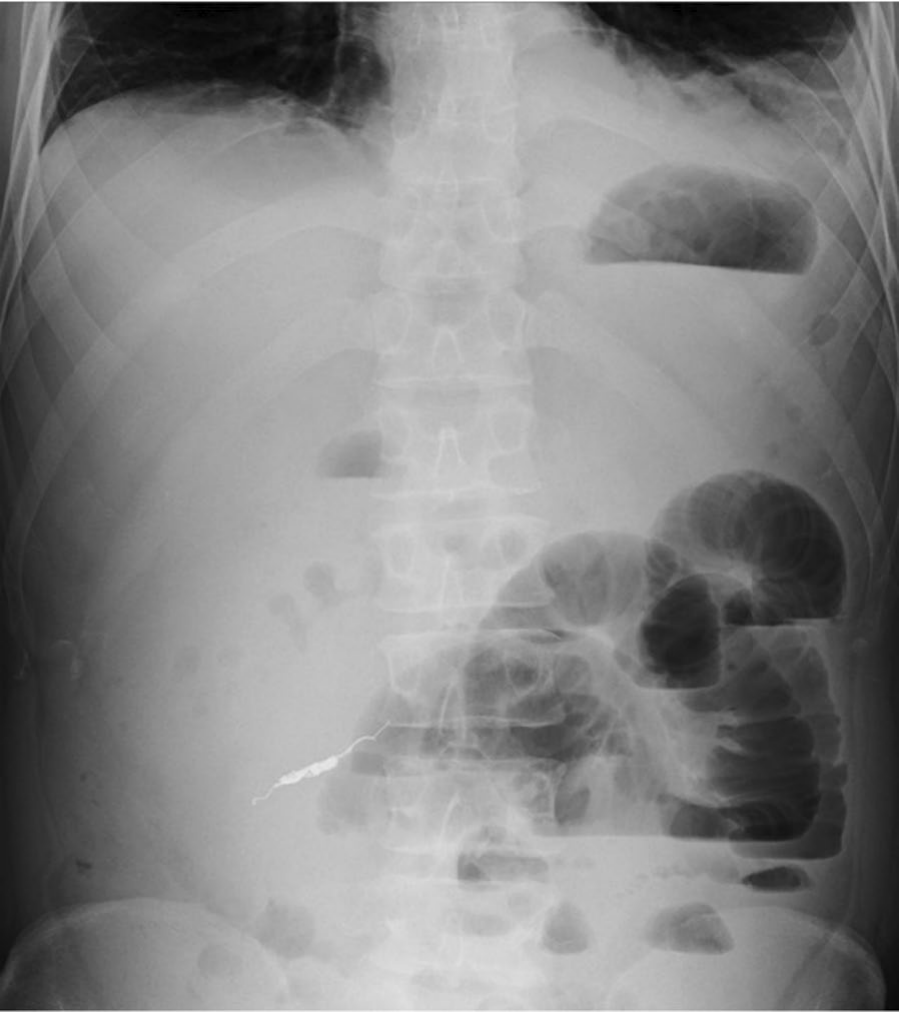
The X-ray shows the dilation and the niveau formation of the small bowel
